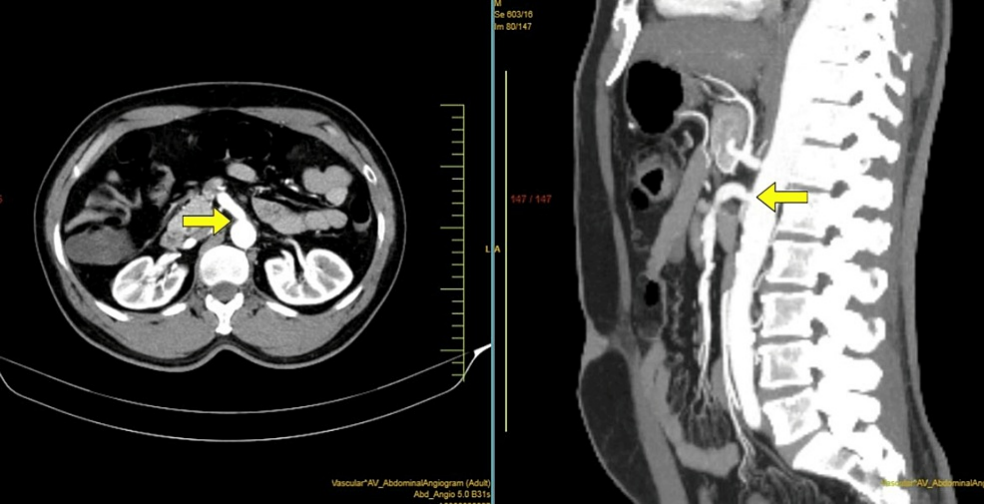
CT aortogram displaying superior mesenteric artery ostial stenosis (yellow arrow)
